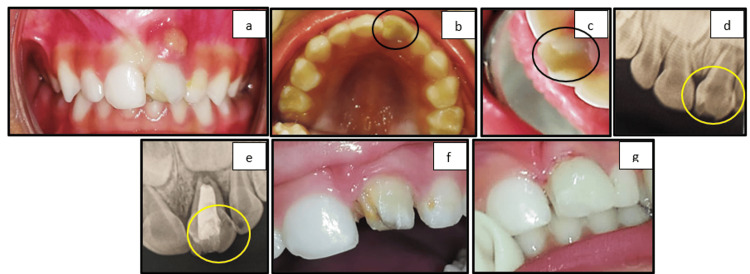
(a) Frontal view showing gingival abscess with respect to 61. (b) Maxillary occlusal view showing talon's cusp with respect to 61. (c) Conical projection over the cingulum region of 61. (d) Preoperative radiograph showing radiolucency in the periapical region of 61. (e) Postoperative radiograph showing the obturated root canal of 61. (f) Crown preparation of 61. (g) Postoperative frontal view showing the strip crown with respect to 61
